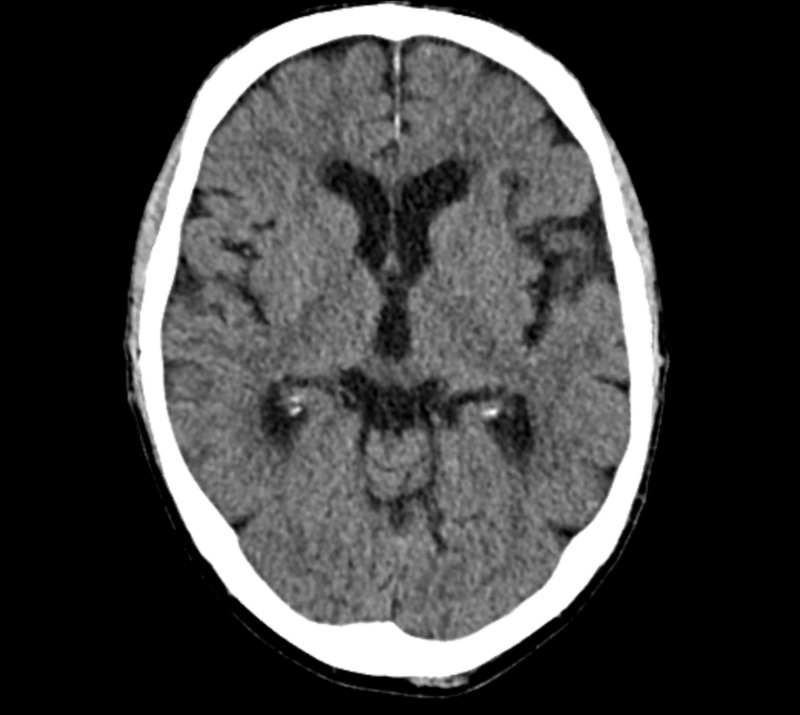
Initial CT of the brain without contrast revealing no acute intracranial abnormality
